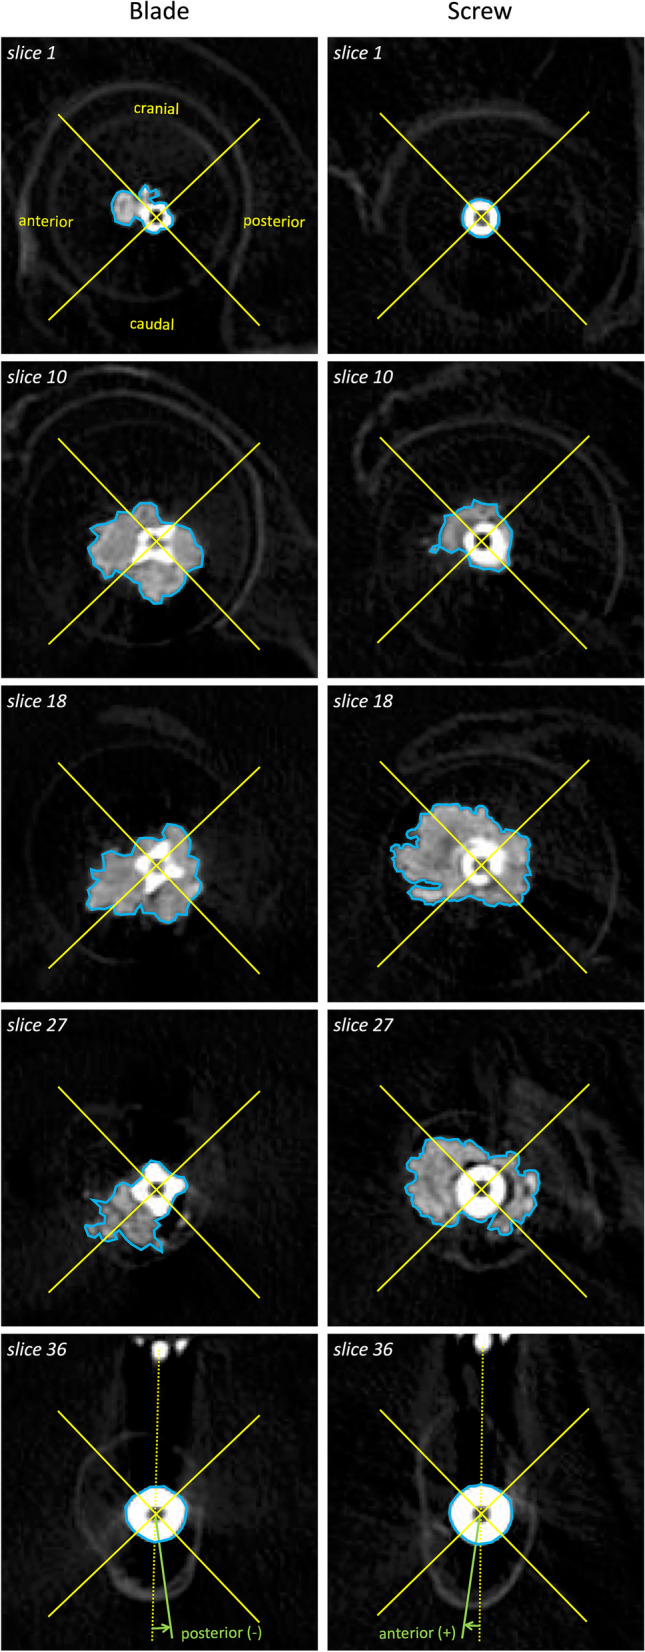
Radiographic parameters using axial CT. In the axial plane, the rotation angle (green arc) was measured on slice 36, which is defined as the angle between the nail–blade line (yellow dotted line) and the blade–medial calcar line (green line). Four directions (cranial, caudal, anterior, and posterior) were defined based on the nail–blade line. On each axial plane through slices 1 to 36, the cross-sectional areas of head elements and dispersed cement (blue polygon shape) were divided into the cranial, caudal, anterior, and posterior parts, and were calculated. In each of the four directions, the sum of cross-sectional areas (total 36 slices) was defined as the volume of the head element. The sum of cement volume in the four directions was defined as the total volume
